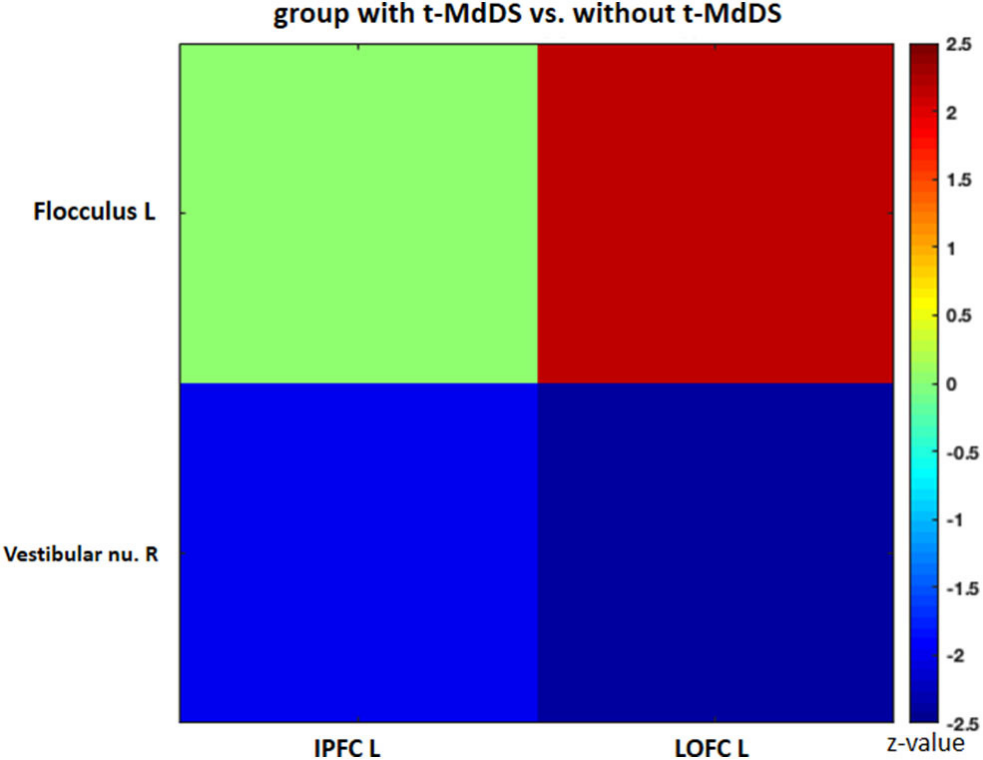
Functional connectivity of the significant regions from the FDG-PET analysis. IPFC, inferior prefrontal cortex; LOPFC, lateral orbital prefrontal cortex; R, right; L, left.
